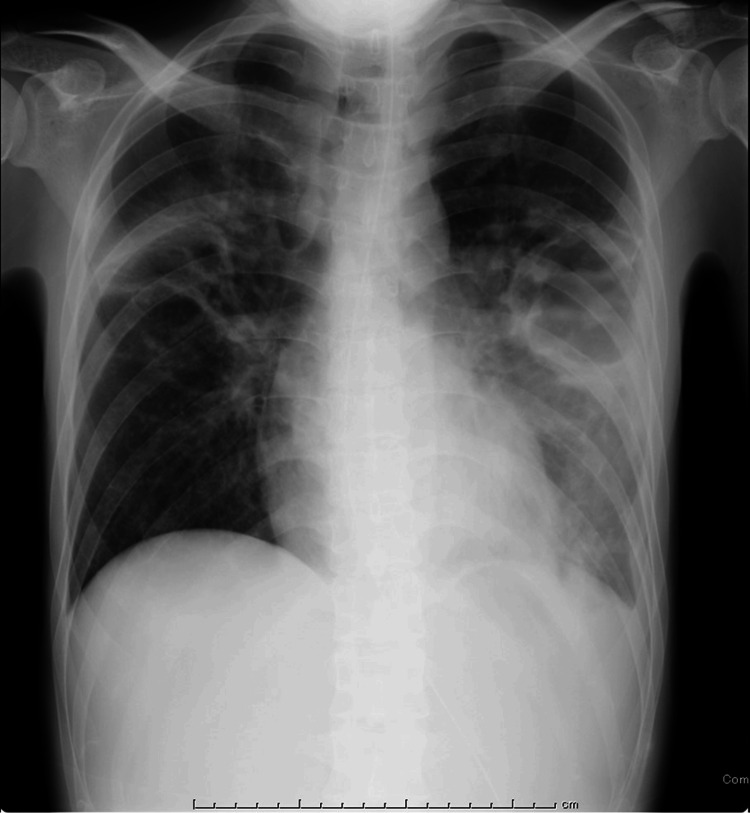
The patient`s chest X-ray The chest X-ray revealed cystic lesions and ground-glass opacities (GGOs) in the left lung field.
